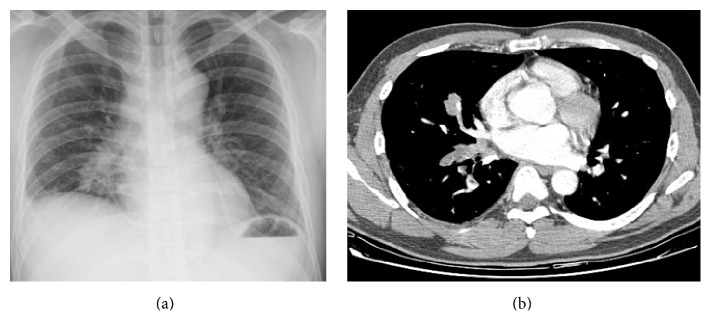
Peripheral thrombotic lesions. The patient is a 41-year-old man with lung cancer. He felt dyspnea. A chest X-ray (a) shows a low right lung lesion. A CT scan (b) shows abnormal tumor/thrombotic lesions within the right superior and right inferior pulmonary veins, with extension into the right lung parenchymal tissue.
